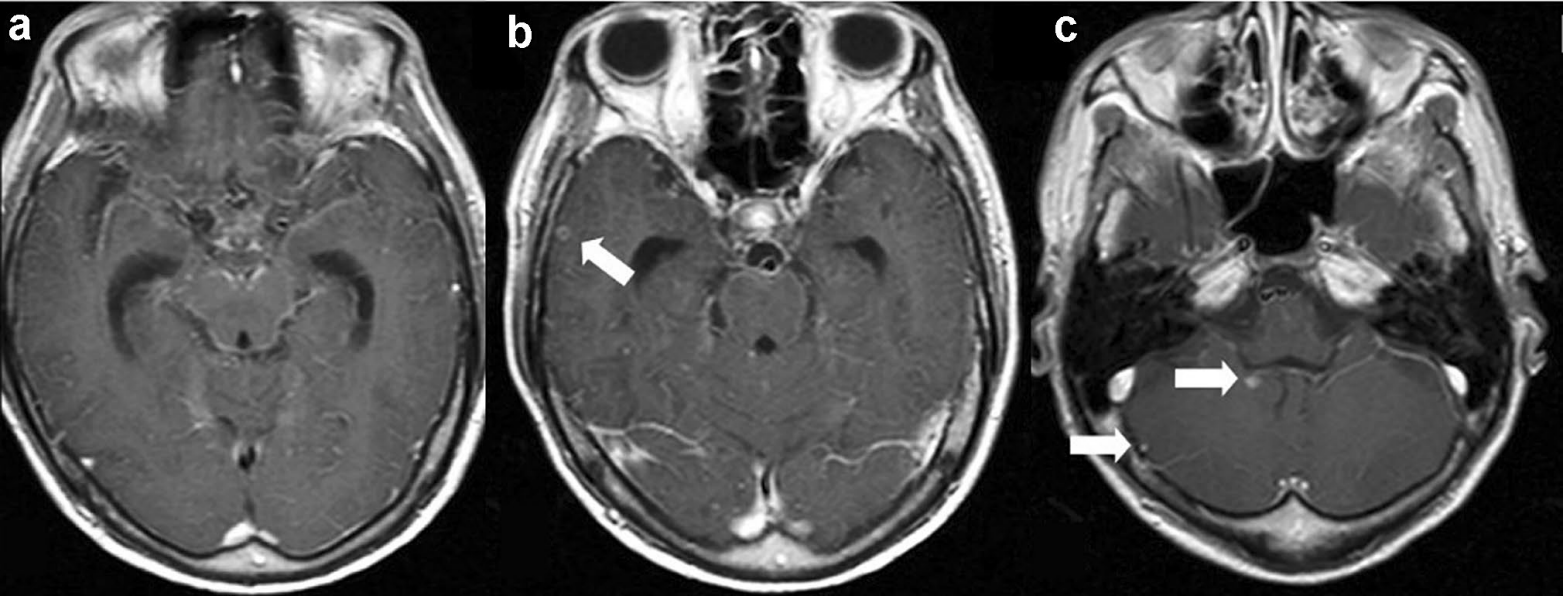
Basilar meningitis and tuberculoma in a patient with HIV-related CNS tuberculosis. Contrast-enhanced T1-weighted image of the basal cistern level (a) shows contrast enhancement along the midbrain, and medial temporal lobe representing meningitis. Peripherally located contrast-enhanced nodules representing tuberculomas are also shown (b, c, arrows)
